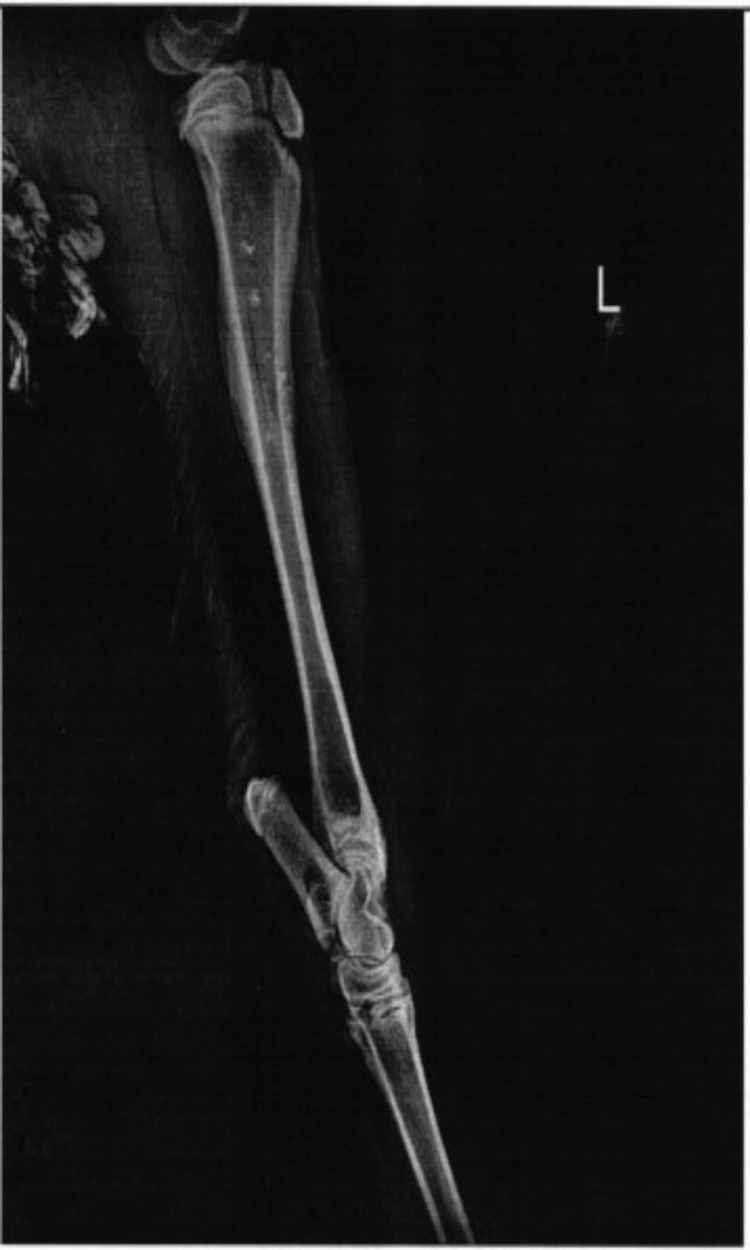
Mediolateral radiographic view 30 days after implantation with mild-to-moderate bone density.
